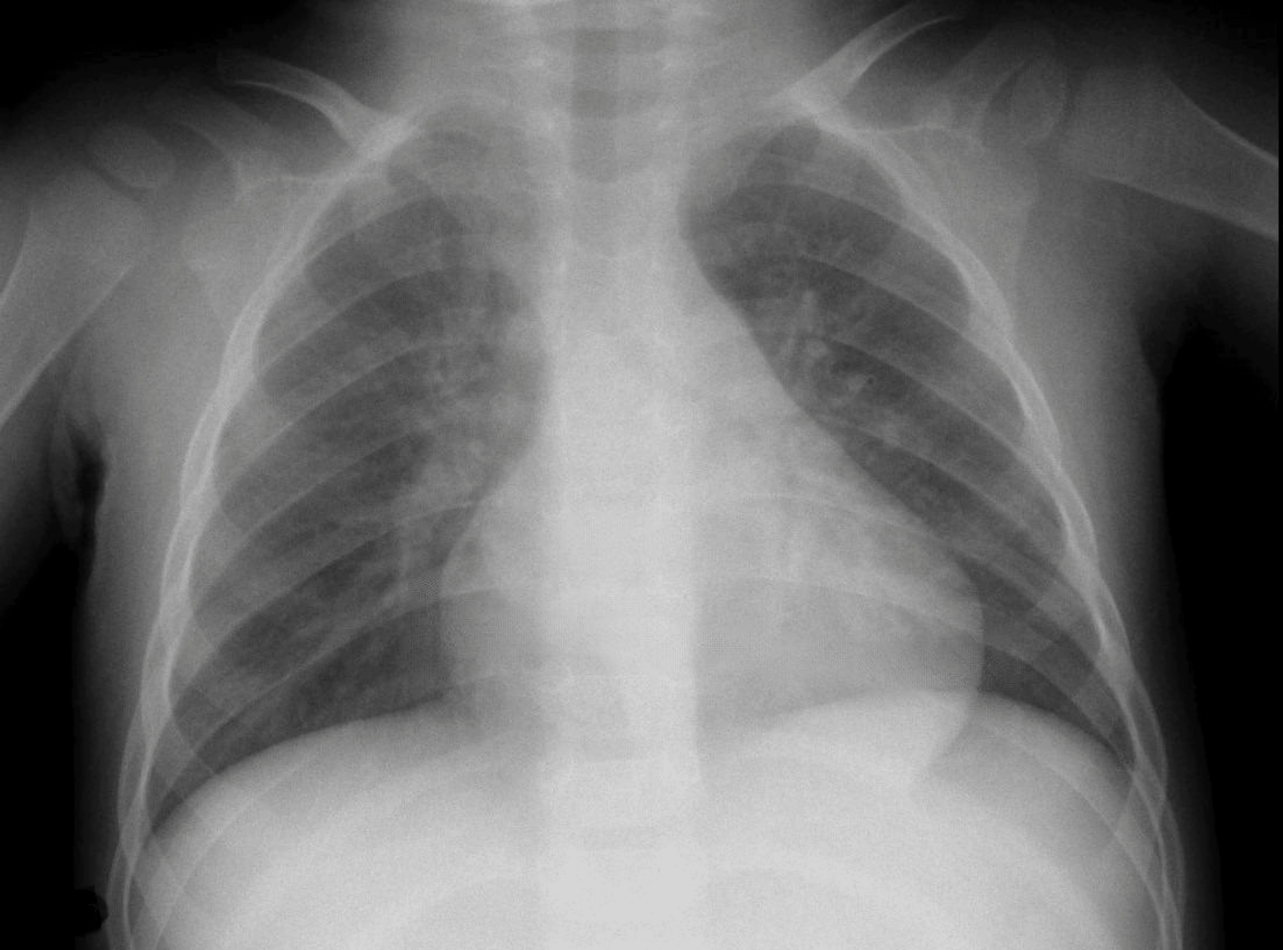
Chest X-ray (anterior-posterior view)
